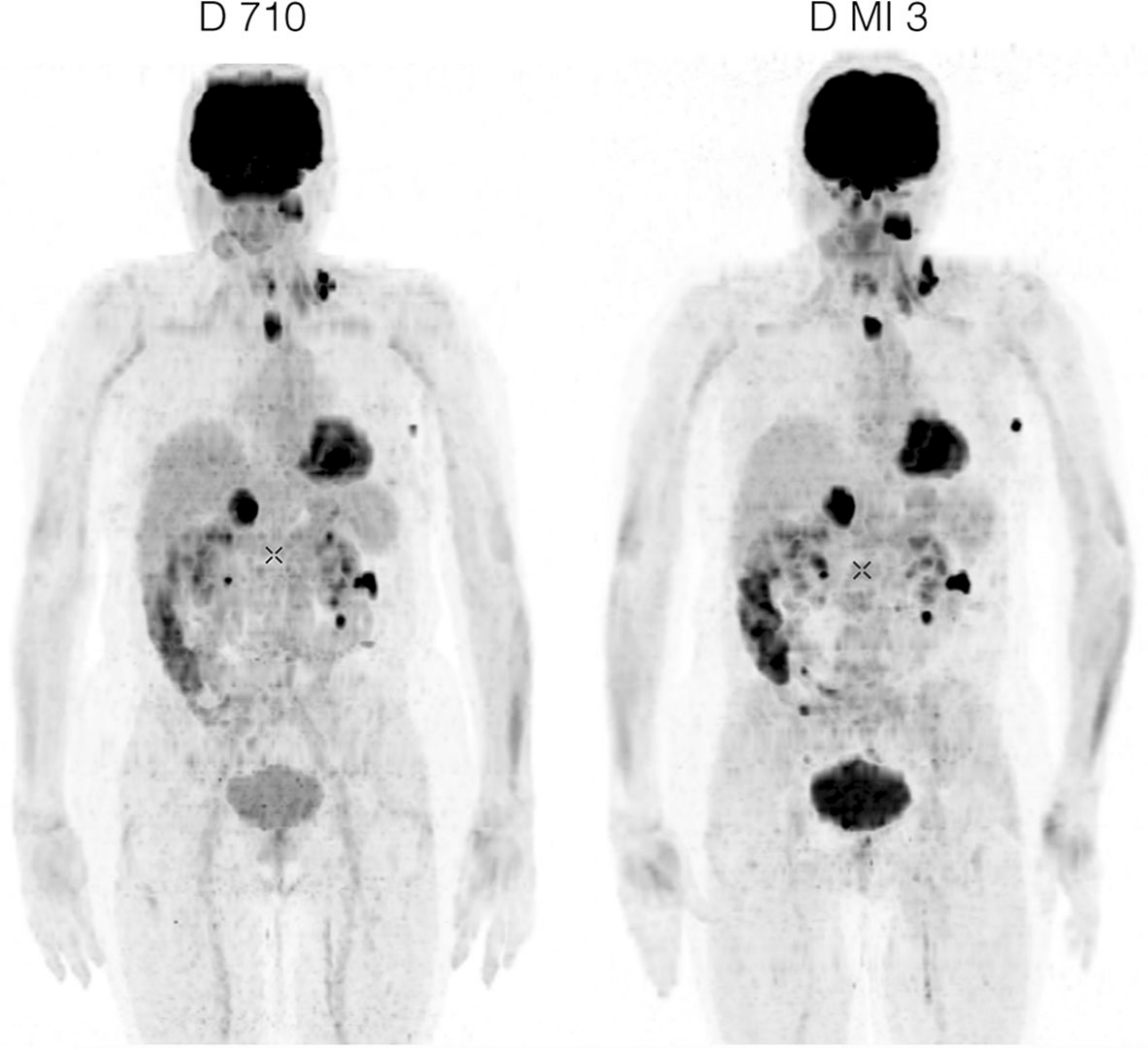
Maximal intensity projection images. These were obtained in a melanoma patient on a Discovery 710 PET/CT (left panel) and, after one half-life radioactive decay, on a Discovery MI 3 PET/CT (right panel), using the same acquisition parameters. Image reconstruction by the Q.Clear algorithm used a beta value of 400 for the Discovery 710 acquisition and 1000 for the MI 3 acquisition. All lesions visible on the Discovery 710 study are visible on the Discovery MI 3 study as well
